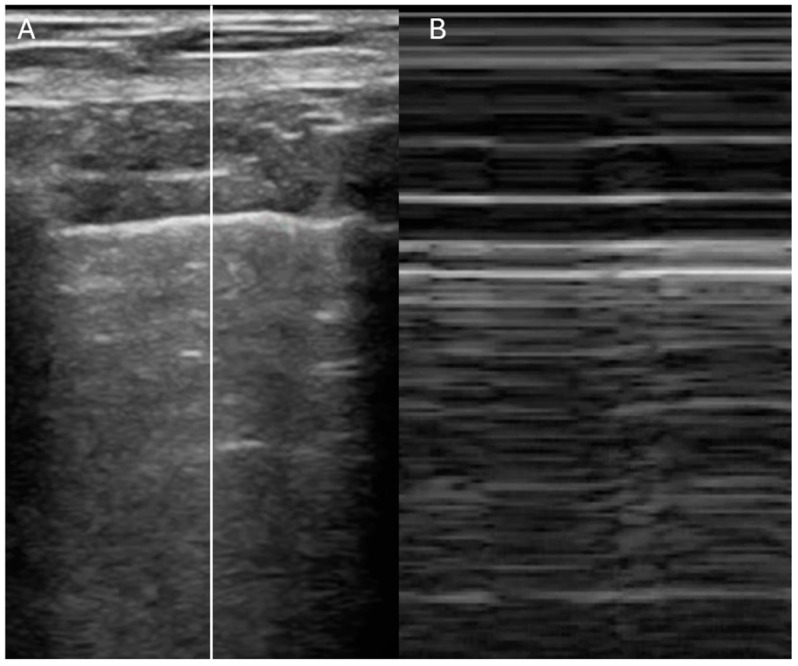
Lung ultrasound showing absent pleural sliding. (A) B-mode. (B) M-mode showing “barcode sign”.
